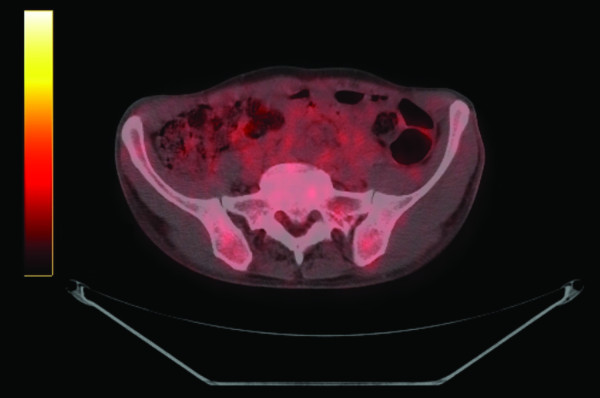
Positron emission tomographic scan. The left pleural mass showed only diffuse low-level fluorodeoxyglucose (^18^F-FDG) uptake of the mass. However, there was a focus of moderate ^18^FFDG activity in the superior aspect of the lesion, which was worrisome for malignancy.
